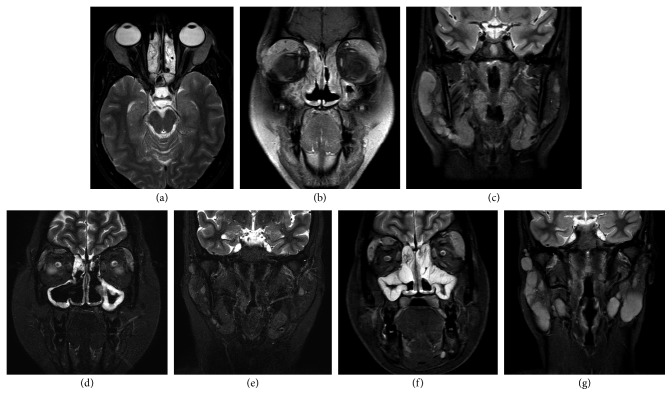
(a, b) MRI head and neck done in May 2013 showing diffuse lacrimal gland enlargement and features of sinusitis. (c) MRI head and neck done in May 2013 showing bilateral parotid and submandibular gland enlargement. (d, e) Follow-up MRI in August 2014 showing significant regression in pan sinusitis, regression in the lacrimal gland, and the salivary gland enlargement after treatment with steroid and azathioprine. (f, g) Follow-up MRI in February 2017 showing disease progression despite steroid and azathioprine.
